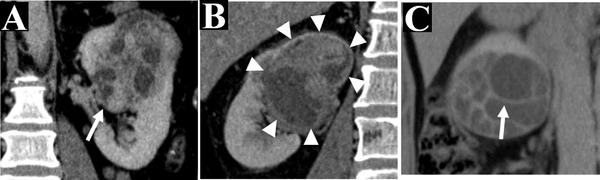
Some vital imaging features on multidetector computed tomography (MDCT). Renal sinus fat invagination (SFI) (white arrow) is shown on the sagittal MDCT (a, case 7), which represents the tumor invasion into the sinus fat (white arrow). In addition, the capsule of the tumor (white triangle) is shown on a coronal MDCT (b, case 12), and the multiple regular septa (white arrow) is shown in the tumor in the coronal MDCT (c, case 11)
